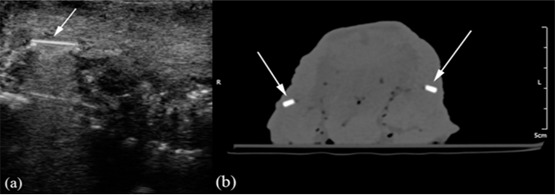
a: Arrow points to a glass foreign body on the ultrasound image. b: Arrows indicate two glass foreign bodies on the CT image.
